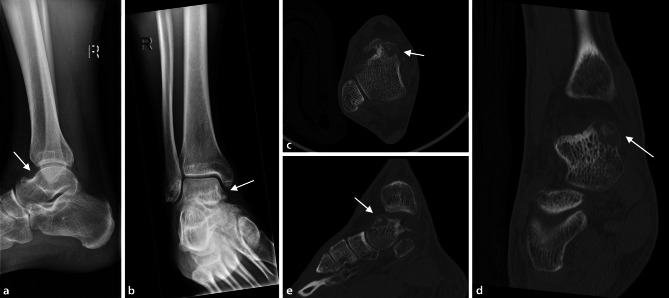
a, b 18-year-old male patient with osteoid osteoma located in the talar neck of the right foot adjacent to the medial talar shoulder, presenting as blurred, lucent region on the anteroposterior (ap) (a) and lateral (b) view (*arrows* pointing at the regions of interest). c–e Transverse (c), frontal (d) and sagittal (e) CT scans showing osteolytic lesion with cortical thinning and central sclerosis in the medial aspect of the talar neck (*arrows* pointing at the regions of interest)
